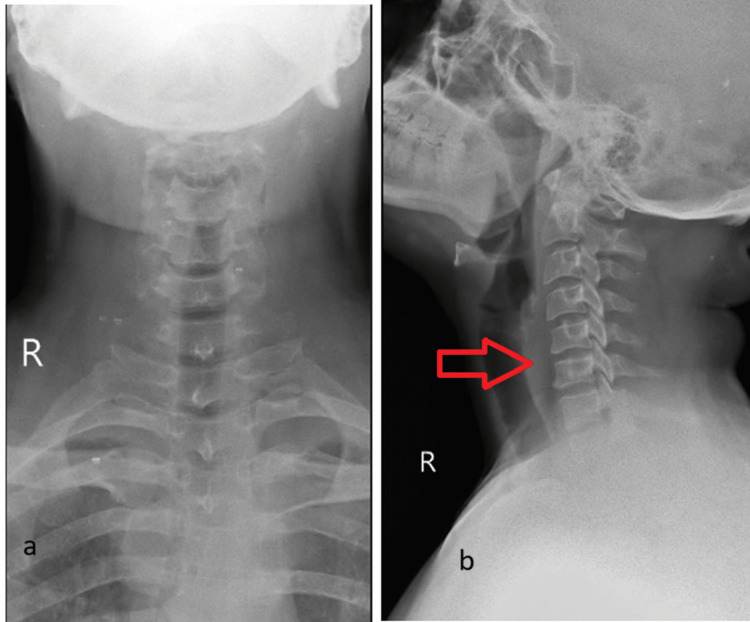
Radiographs of the cervical spine ((a) anteroposterior and (b) lateral views) showing bridging syndesmophytes, loss of cervical lordosis, and reduced space between C5 and C6 (arrow)
